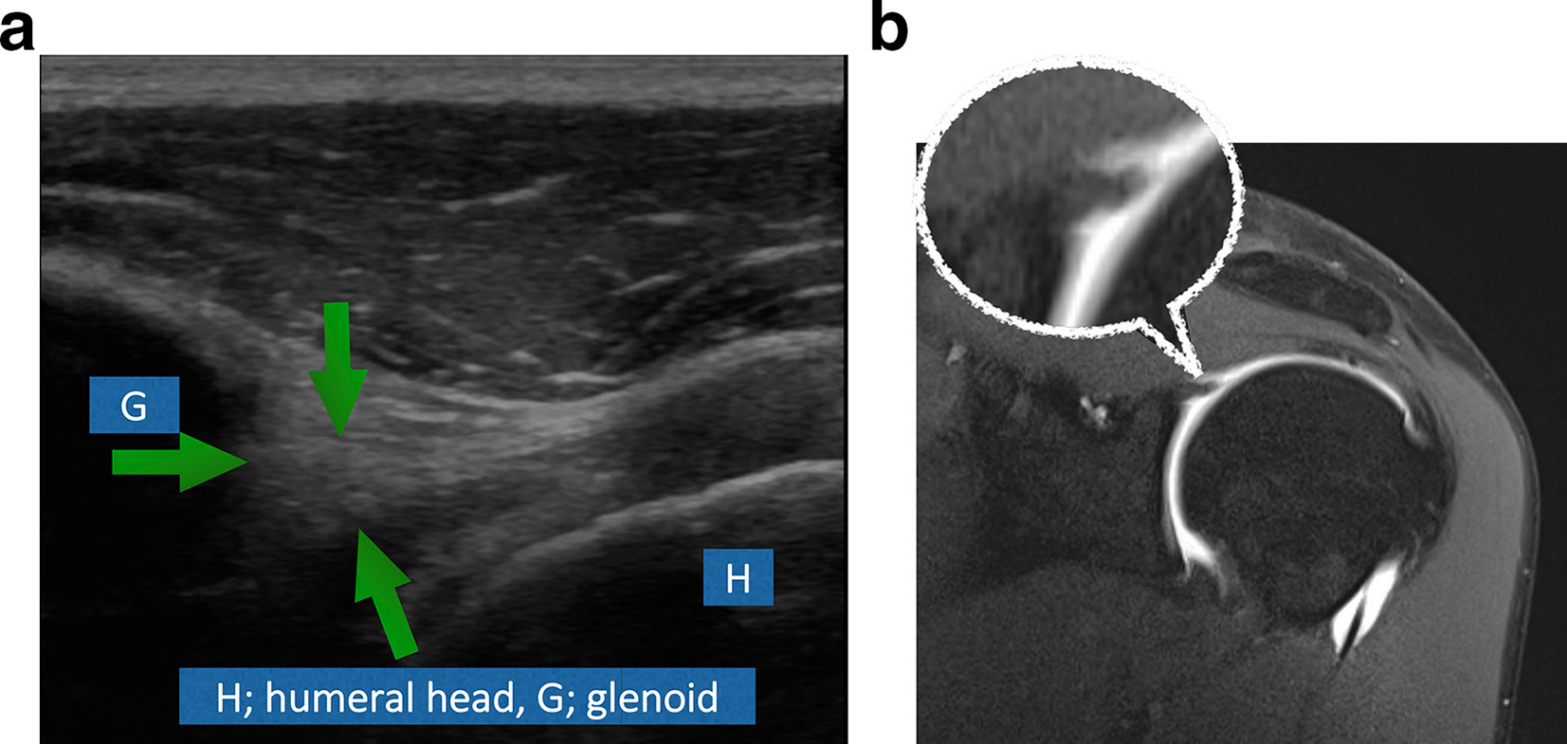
(A) A 40-year-old male patient who came with a history of recurrent subluxation. His MRI shoulder (Figure 3b) shows a normal superior labrum similar to the ultrasound findings (Figure 3A). The superior labrum appears as an echogenic triangular structure on ultrasound.
